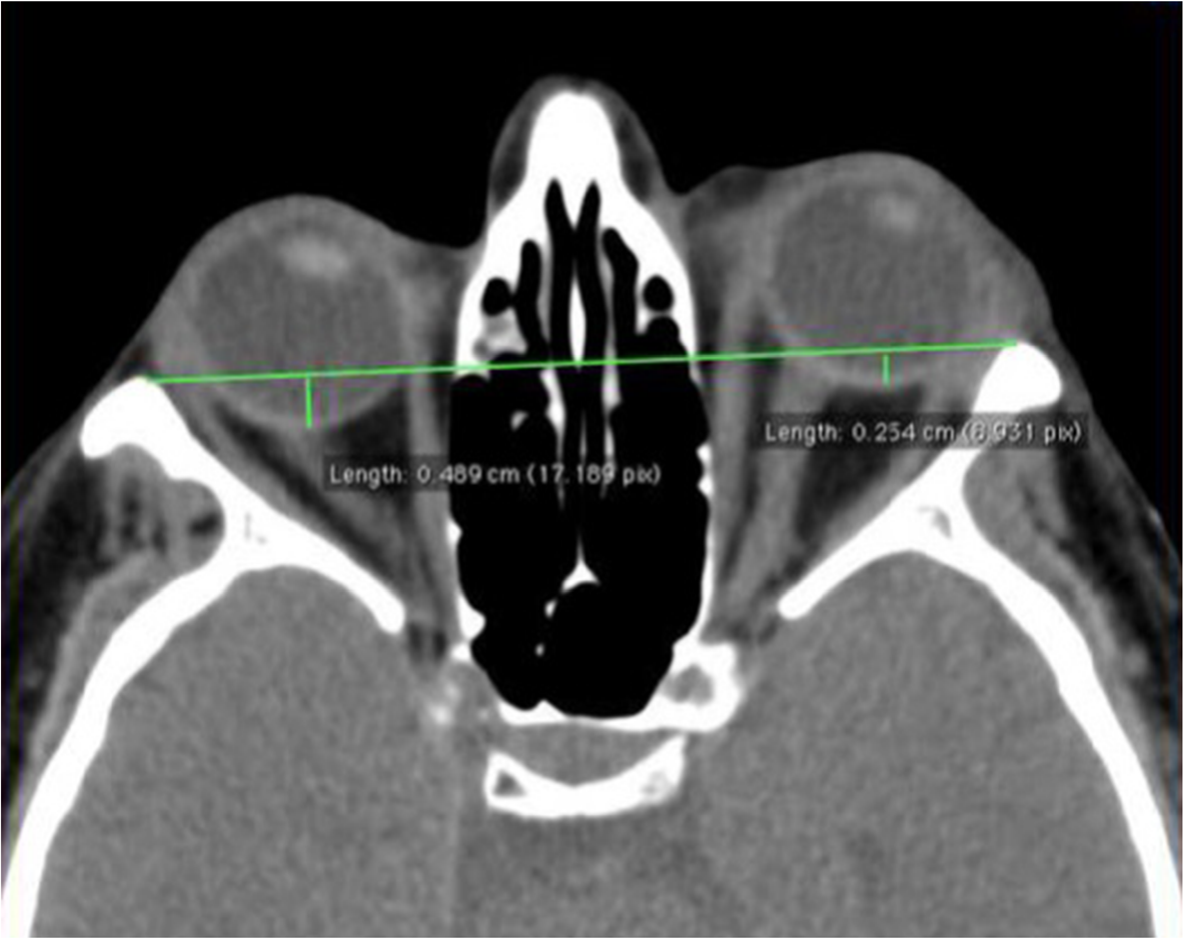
Axial 1.3 mm CT scan of the orbit in soft-tissue windows. Unilateral exophthalmos is evaluated by the perpendicular distance between the interzygomatic line and the posterior margin of the globe at the mid-globe sections.
